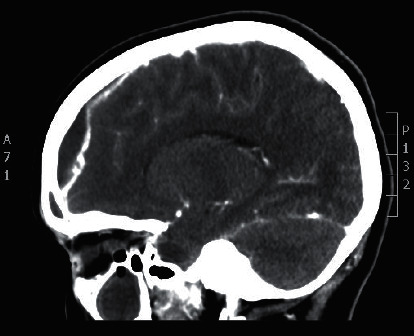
Sagittal brain CT scan showing a subdural empyema.
